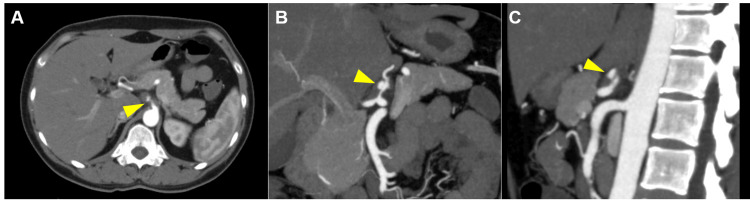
Contrast-enhanced CT of the celiac artery Dissection and narrowing of the celiac artery are seen (yellow arrowheads). A: Axial view, B: coronal view, C: sagittal view.
